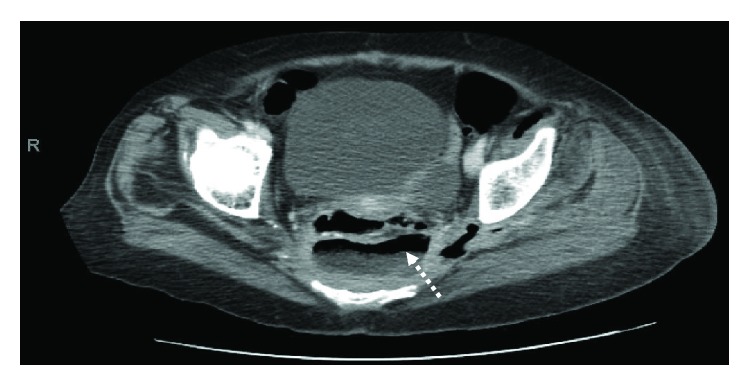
Axial CT images with IV contrast of the pelvis showing the extraperitoneal abscess (dotted arrow) derived from a previous colorectal anastomosis.
